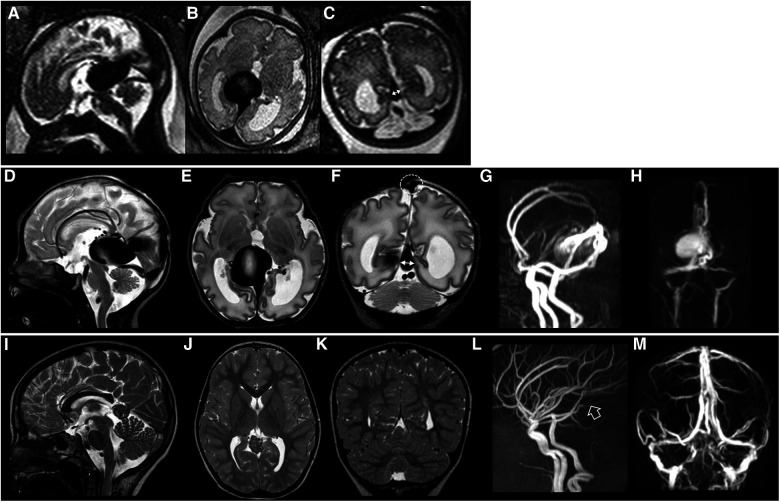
Fetal brain MRI performed at 34 gestational weeks (A–C), post-natal brain MRI performed at birth (D–H), and last brain MRI (I–M) performed at 8 years of age in patient #26 with a good neurological outcome. Fetal and post-natal sagittal (A,D), axial (B,E) and coronal (C,F) T2-weighted images demonstrate a VGAM associated with low SS-MD (thin arrows with double heads, 4.3 mm). Note on post-natal MRI that there is no stenosis of the superior sagittal sinus (dotted circle) with SSS index of 3.3. MR arterial (G) and venous (H) angiography reveal a large VGAM pouch with feeders from the choroidal and anterior cerebral arteries. There is no stenosis of the jugular bulbs. Last brain MRI (I–K) and MR arterial and venous angiography (L,M) show complete occlusion of the VGAM pouch (empty arrow). No brain complications are evident in this case.
